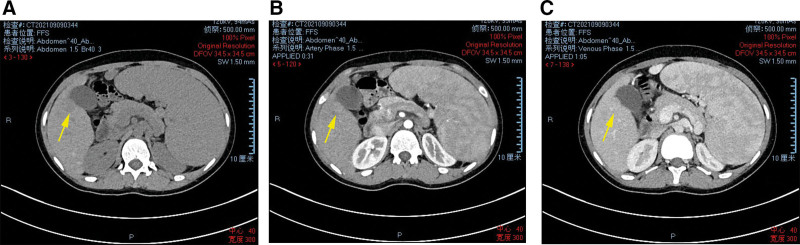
Plain scan and enhanced CT results. The results of plain scan showed that there were many slightly high-density shadows in the hepatic parenchyma (A), and the results of contrast-enhanced scan showed slight enhancement in arterial phase (B) and slight regression in portal phase (C).
